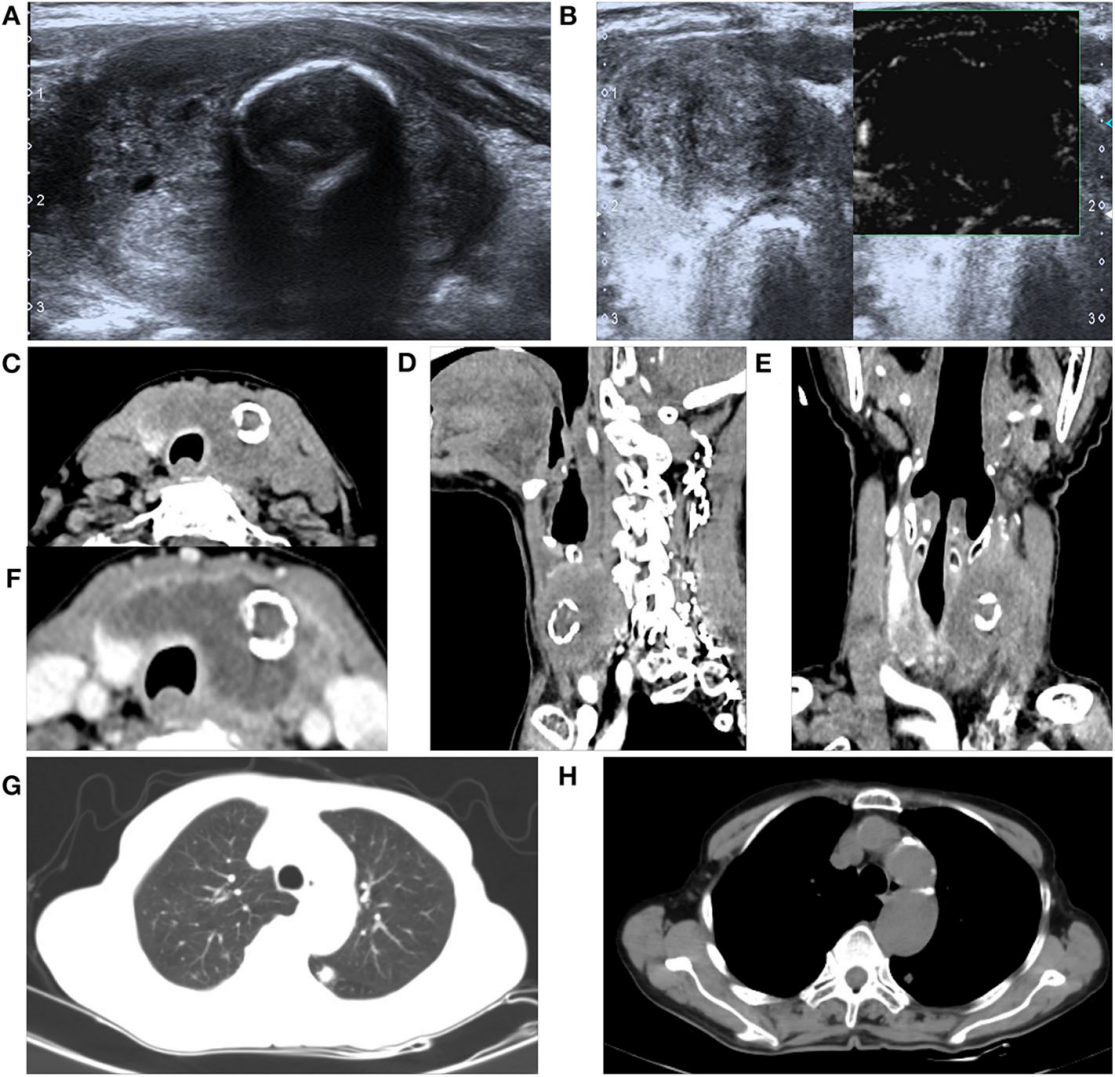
A 76-year-old female with primary squamous cell carcinoma of the thyroid (PSCCT) in the left neck. (A) Low signal of grayscale ultrasound shows heterogeneously hypoechoic solid mass sized about 5 × 4 × 3 cm in her left thyroid gland accompanied with a 1.5 × 1.2-cm-size, well-defined, round-like microcalcification located in the central portion. (B) Preoperative fine-needle aspiration cytology of the mass shows no definite signs of malignancy. (C–E) CT scans show an annular calcification accompanied with a peripheral cystic degeneration portion in her left thyroid gland accompanied with the right deviation of trachea (axial, sagittal, and coronal planes). (F) Contrast-enhanced CT shows obvious heterogeneous enhancement with the central non-enhancing necrotic portion, and the thyroid capsule is intact. Chest CT suggested small nodules in the lower lobe of left lung (G) pulmonary window and (H) mediastinal window.
